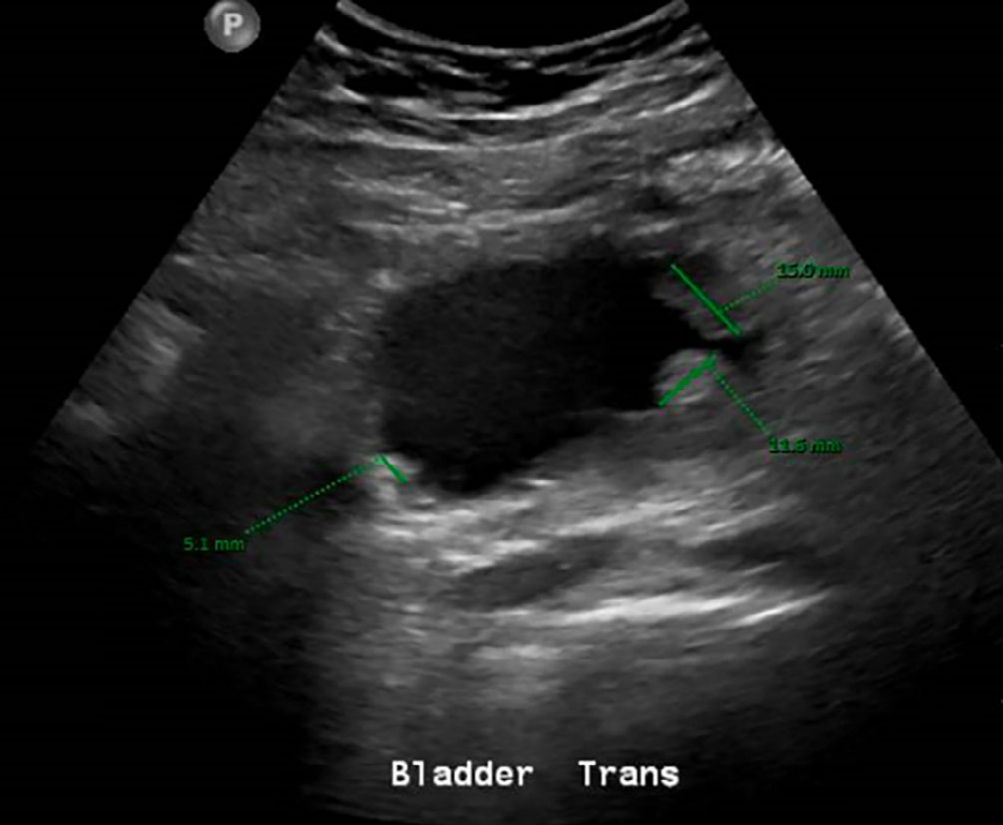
Renal ultrasound. Three urinary bladder mucosal polyploidal masses measuring 1.5 × 0.9, 1, and 0.5 cm were observed, highly suspicious for primary urothelial neoplasms.
